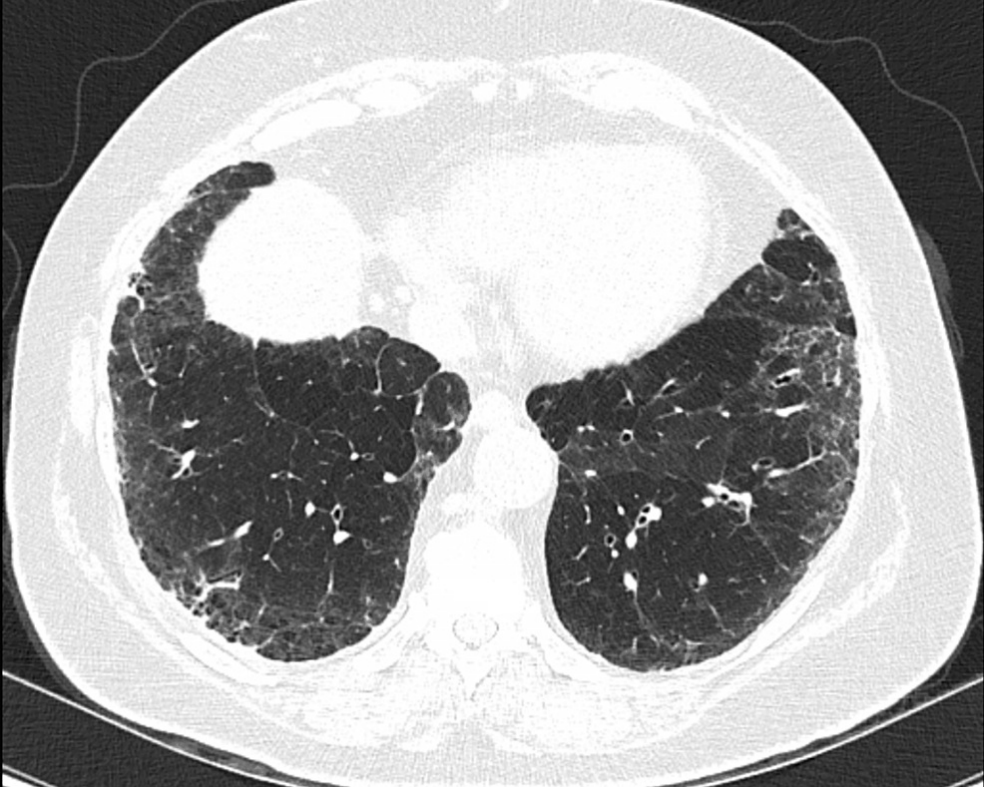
CT Chest showing worsening reticular changes, honeycombing, and traction bronchiectasis in the lower lobes
